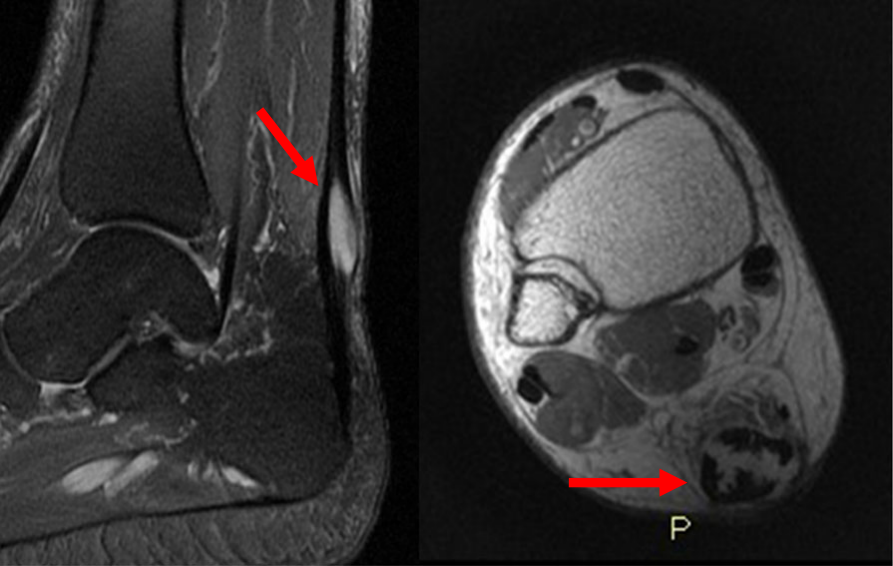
Pre-operative MRI showed a mass in the context of the middle third of Achilles tendon, with hyperintense signal in T2-weighted and T2-weighted fat-sat images
